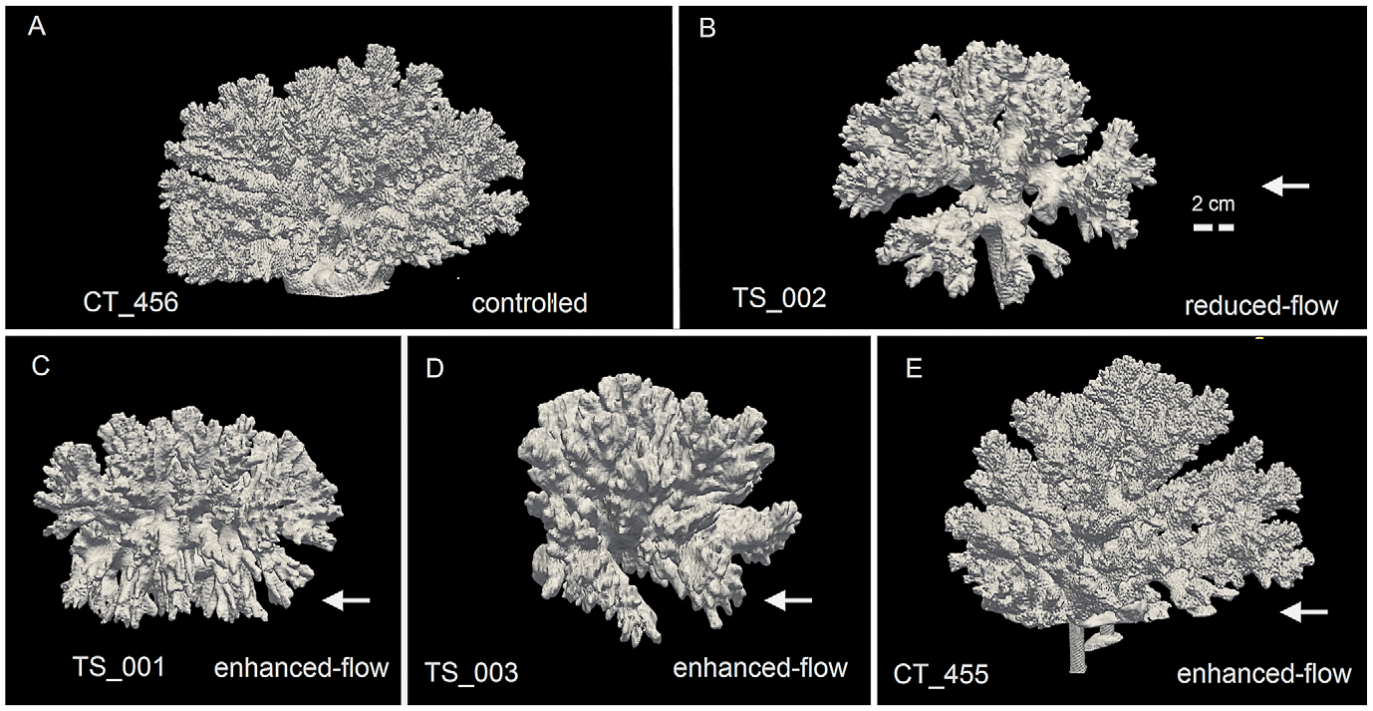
Computed Tomography (CT) scans of scleractinian coral *Pocillopora verrucosa*. (A) A controlled coral exposed to ambient current with the average near-bottom velocity of 5 cm s^−1^
